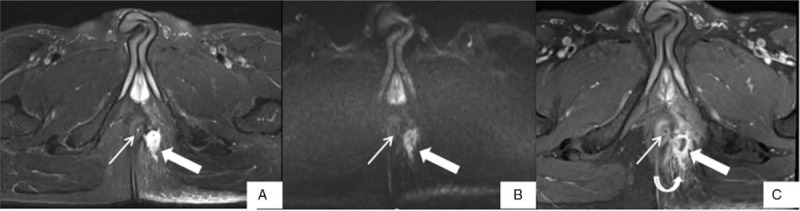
MR images of a 49-year-old man with a transsphincteric perianal fistula. (A) The internal orifice (thin arrow) is identified in the 3 o’clock direction, and the fistula tract (thick arrow) is noted in the left ischioanal fossa on axial fat suppressed T2-weighted imaging (FS T2WI). (B) The internal orifice (thin arrow) is almost invisible on axial diffusion-weighted imaging (DWI), and the fistula tract (thick arrow) is noted in the left ischioanal fossa. (C) The internal orifice (thin arrow) is identified in the 3 o’clock direction, and the fistula tract (thick arrow) is noted in the left ischioanal fossa on axial fat suppressed T1-weighted imaging with contrast enhanced (FS T1WI+CE). It shows not only the enhancement of the fistula tract, but also the enhancement of the inflammatory infection tissue (curved arrow) around the fistula. It is recorded a slightly higher confidence score for lesion conspicuity on FS T2WI than on FS T1WI+CE.
